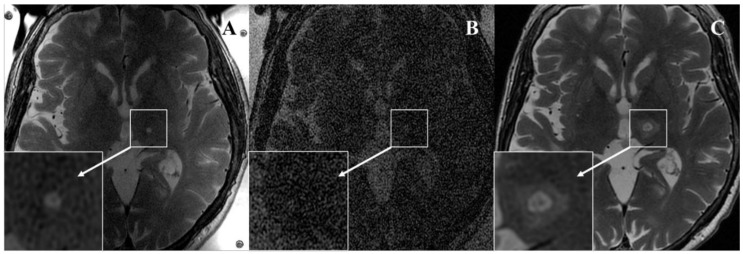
Seventy-eight-year-old woman with essential tremor. (A) Intraoperative axial 2D FRFSE T2-weighted sequence acquired after the last high-energy sonication using the dedicated two-channel FUS-Head coil well demonstrates the presence of zone I (hypointense) and zone II (hyperintense) in the left nucleus ventralis intermedius. (B) Intraoperative axial 2D FRFSE T2-weighted sequence acquired using body radiofrequency coil does not visualize any lesional zones. (C) Forty-eight-hour follow-up acquired with the eight-channel phased-array head coil demonstrates enlargement of zone I and zone II and development of peripheral vasogenic edema (zone III). Both readers agreed on the presence of these imaging findings.
